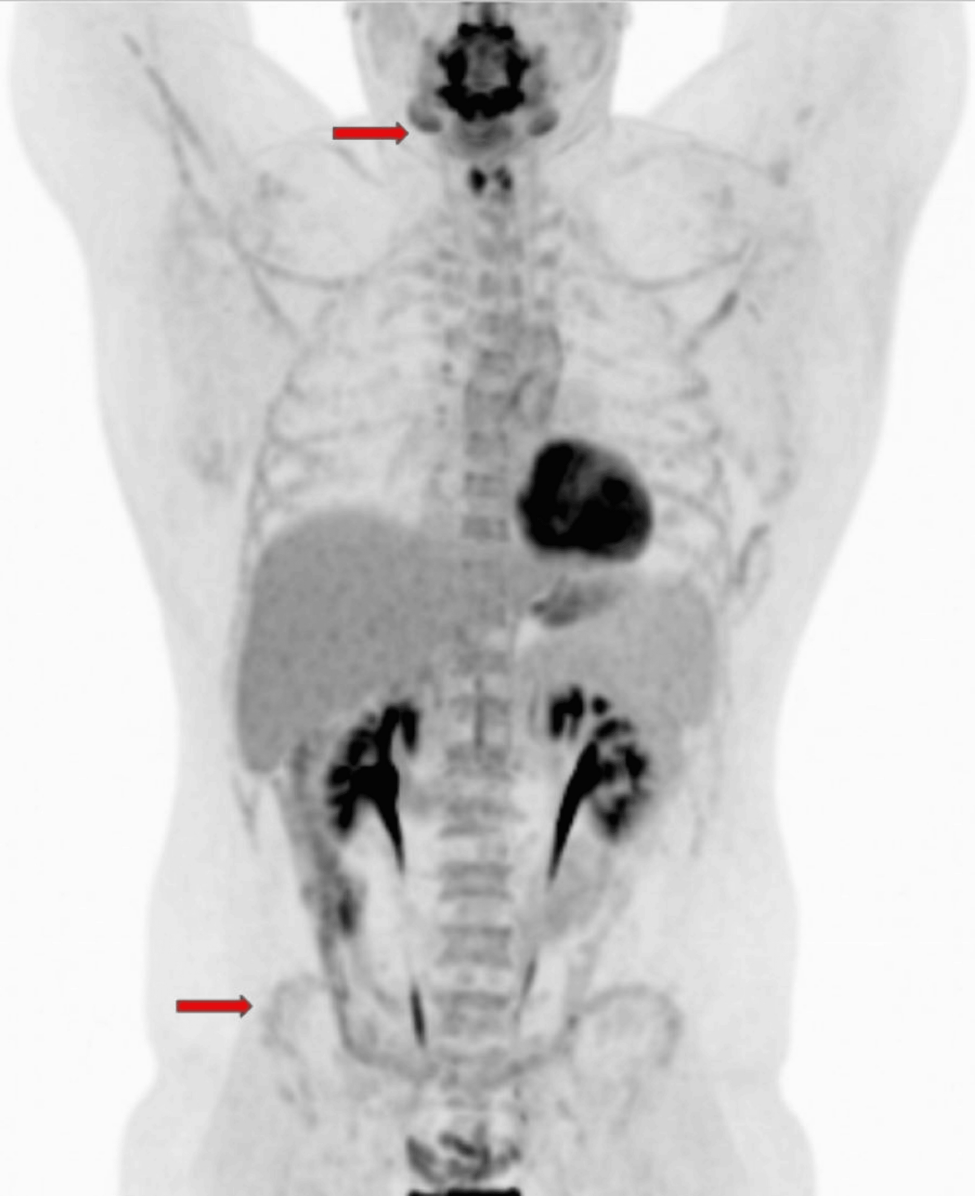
PET/CT showed a decrease in FDG avidity in the proximal appendicular skeleton. There is no FDG-avid in the cervical lymph nodes or thyroid nodule. PET/CT= Positron emission tomography/computed tomography scan, FDG=fluorodeoxyglucose
